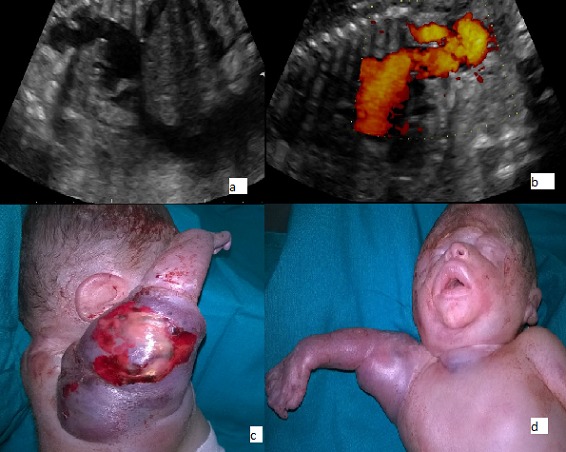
(A, B) doppler énergie montrait le départ de vaisseaux de l’aorte communiquant avec l’artère sous clavière droite; (C, D) tumeur vascularisée prenant et déformant tout le membre supérieur droit, allant jusqu’à la partie haute du thorax
